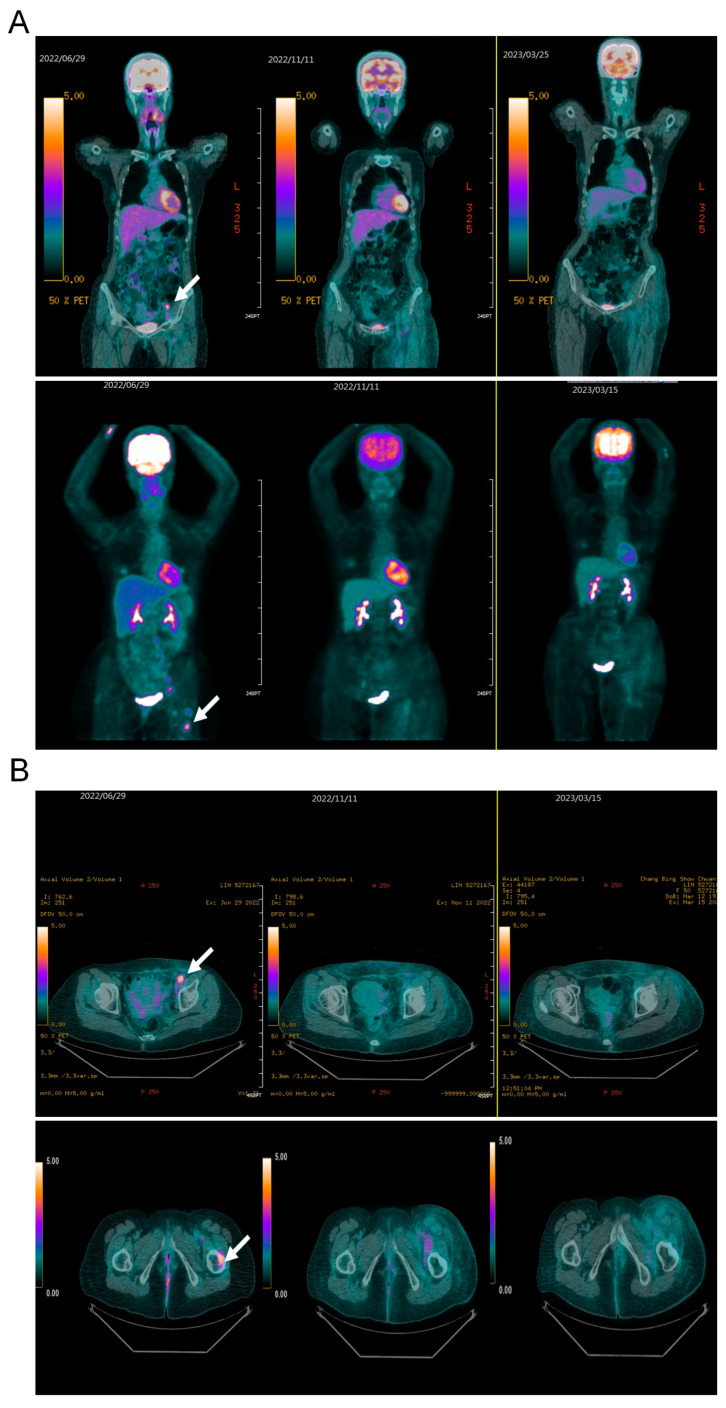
Response to treatment in a patient with endometriosis-associated clear cell carcinoma, presenting with left inguinal lymph node and left femoral bone metastases. (A) 3D maximum intensity projection of 18F-FDG-PET scans (anterior views) conducted before treatment (June 2022), 5 months after treatment initiation (November 2022), and 9 months into treatment (March 2023). The pretreatment images show multifocal hypermetabolic foci (indicated by arrows), with the most intense and notable activity in the left inguinal lymph node and left upper femoral bone. (B) A selected transaxial slice showing increased FDG uptake in the left inguinal lymph node and left upper femoral bone.
